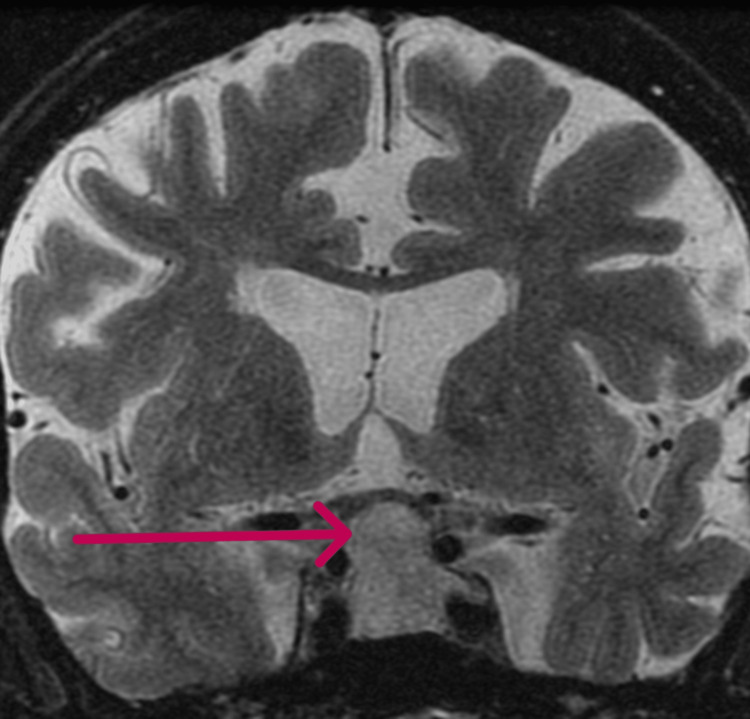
MRI image of the pituitary gland The arrow shows a large pituitary tumor pushing on the optic chiasma.
